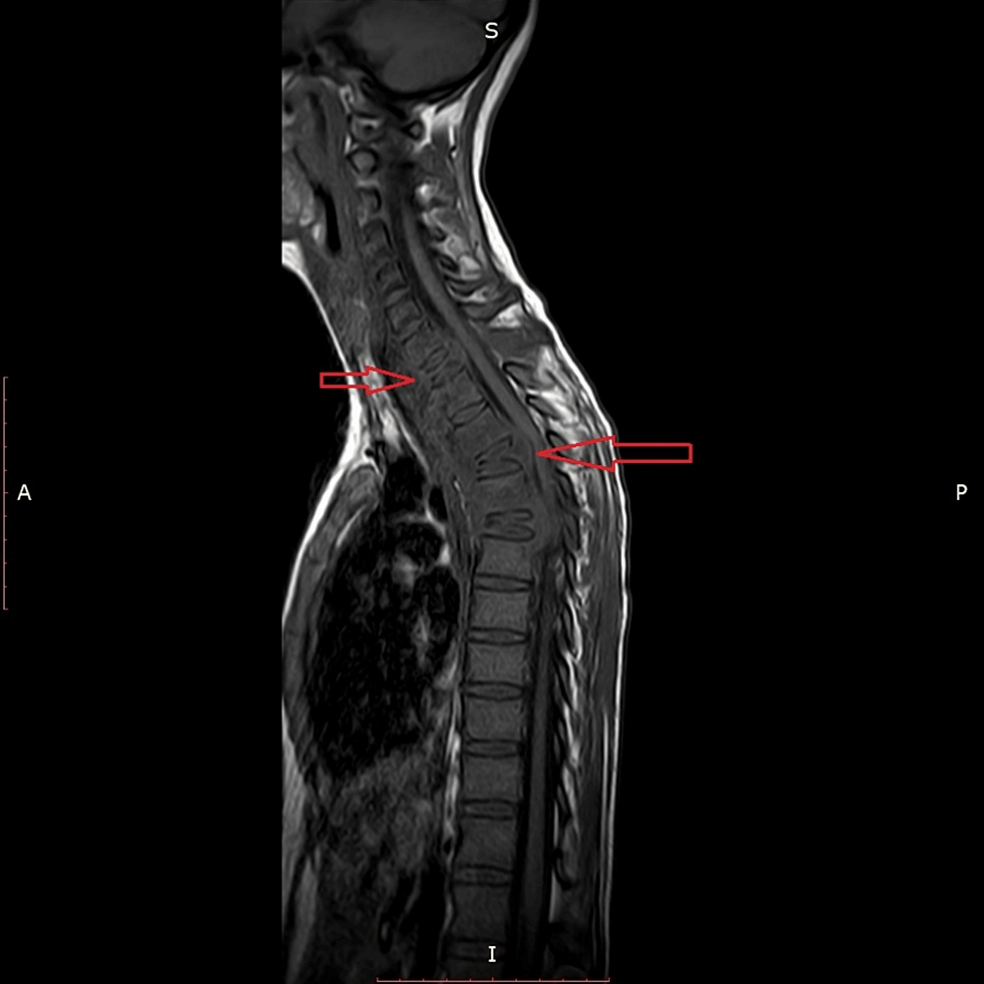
MRI cervical-dorsal spine eT1W showing compression collapse of the D1, D4, AND D6 vertebral bodies. The signal intensities appear hypointense.
